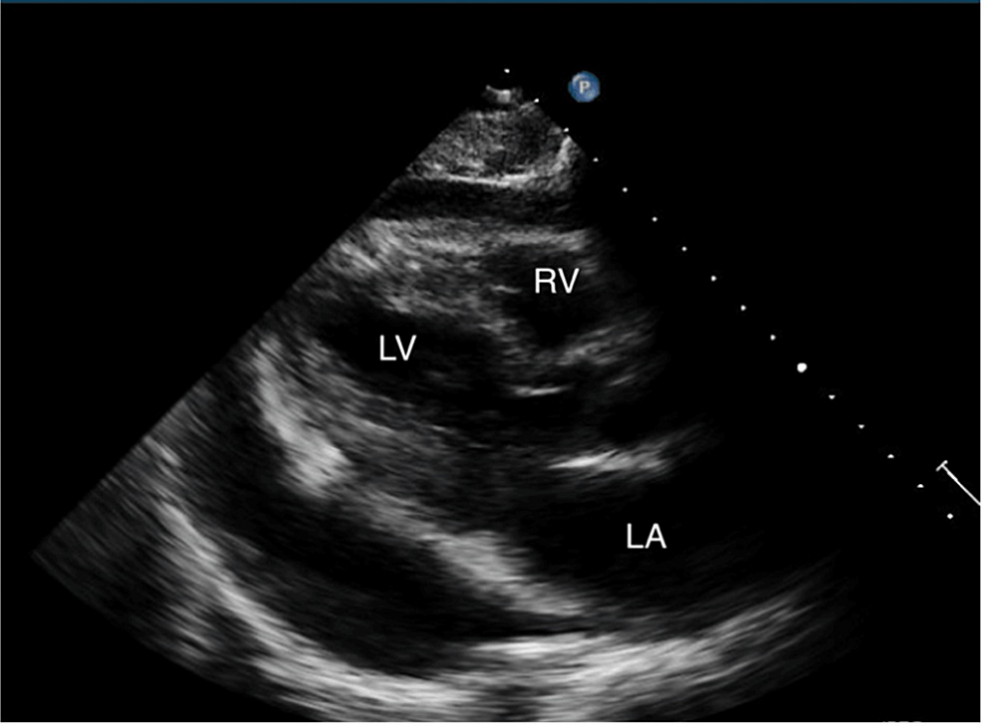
Transthoracic echocardiogram depicting a large pericardial effusion.
